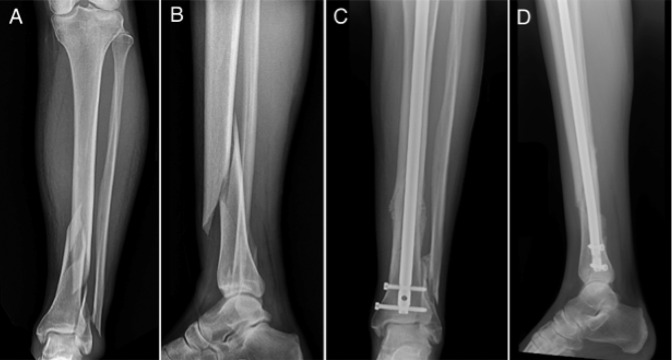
Radiograph demonstrating the (A) AP and (B) lateral injuries of an extra-articular distal tibial fracture and (C) AP and (D) lateral radiographs following intramedullary nail fixation.
